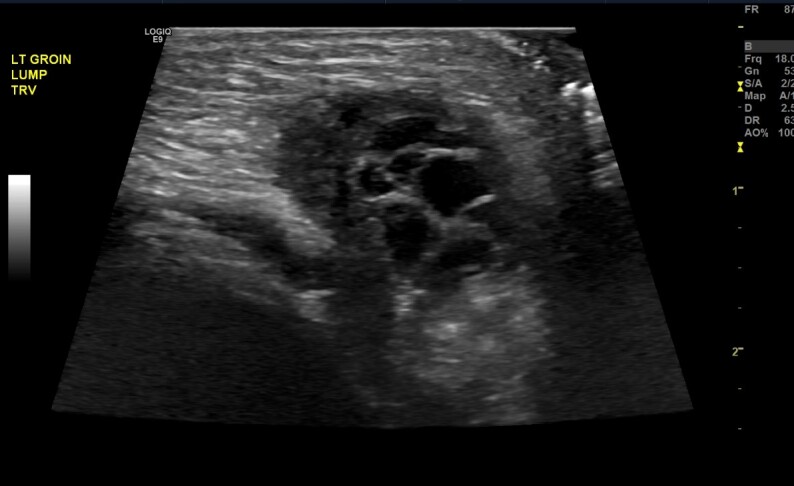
Ultrasonography (transverse view) showing an incarcerated ovary (arrow) within an inguinal hernia.
